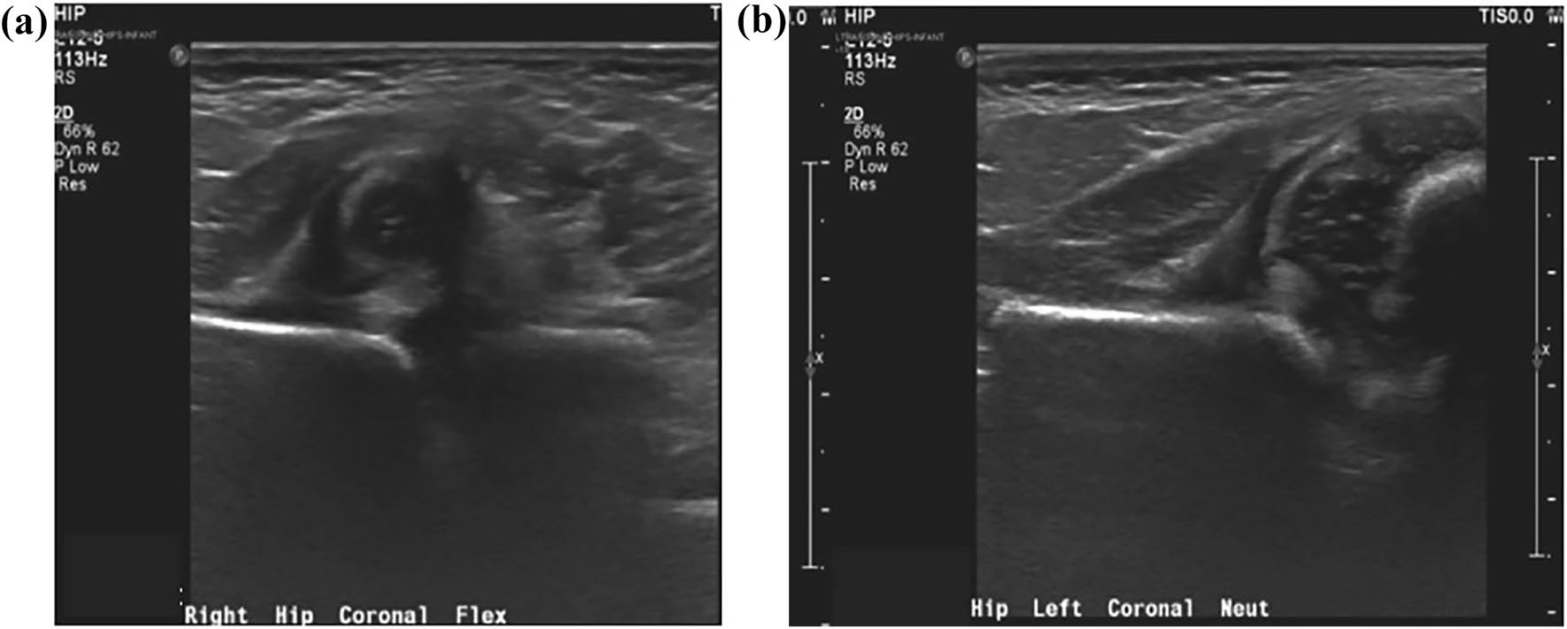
Coronal right hip (a) and left hip (b) ultrasound images of an 8-week-old girl with bilaterally frankly dislocated hips. This girl failed the 3-week Pavlik harness treatment. At 12 weeks of age, closed reduction was successful with adductor tenotomy and arthrography
